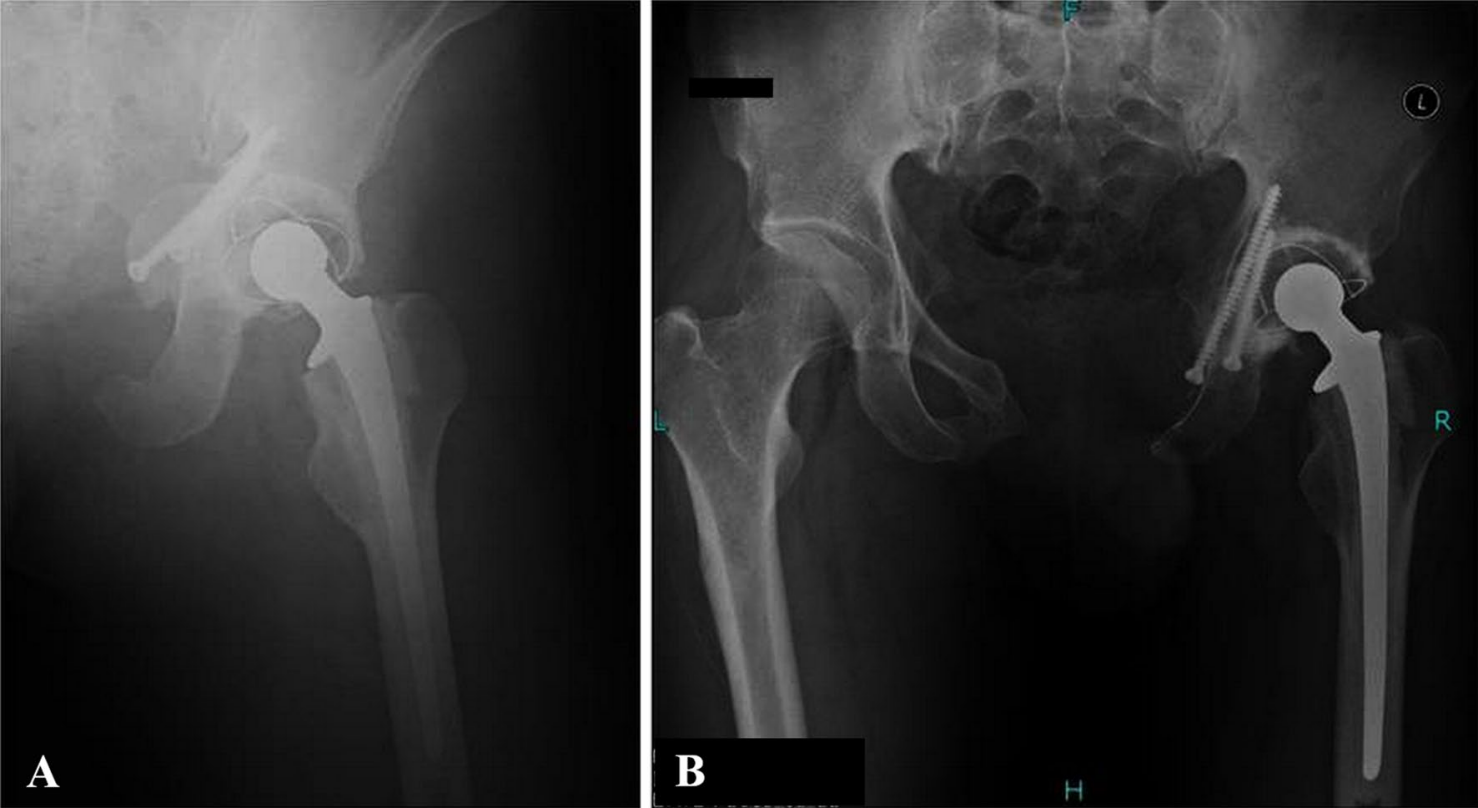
The radiography postoperative and 50 months postoperative.
